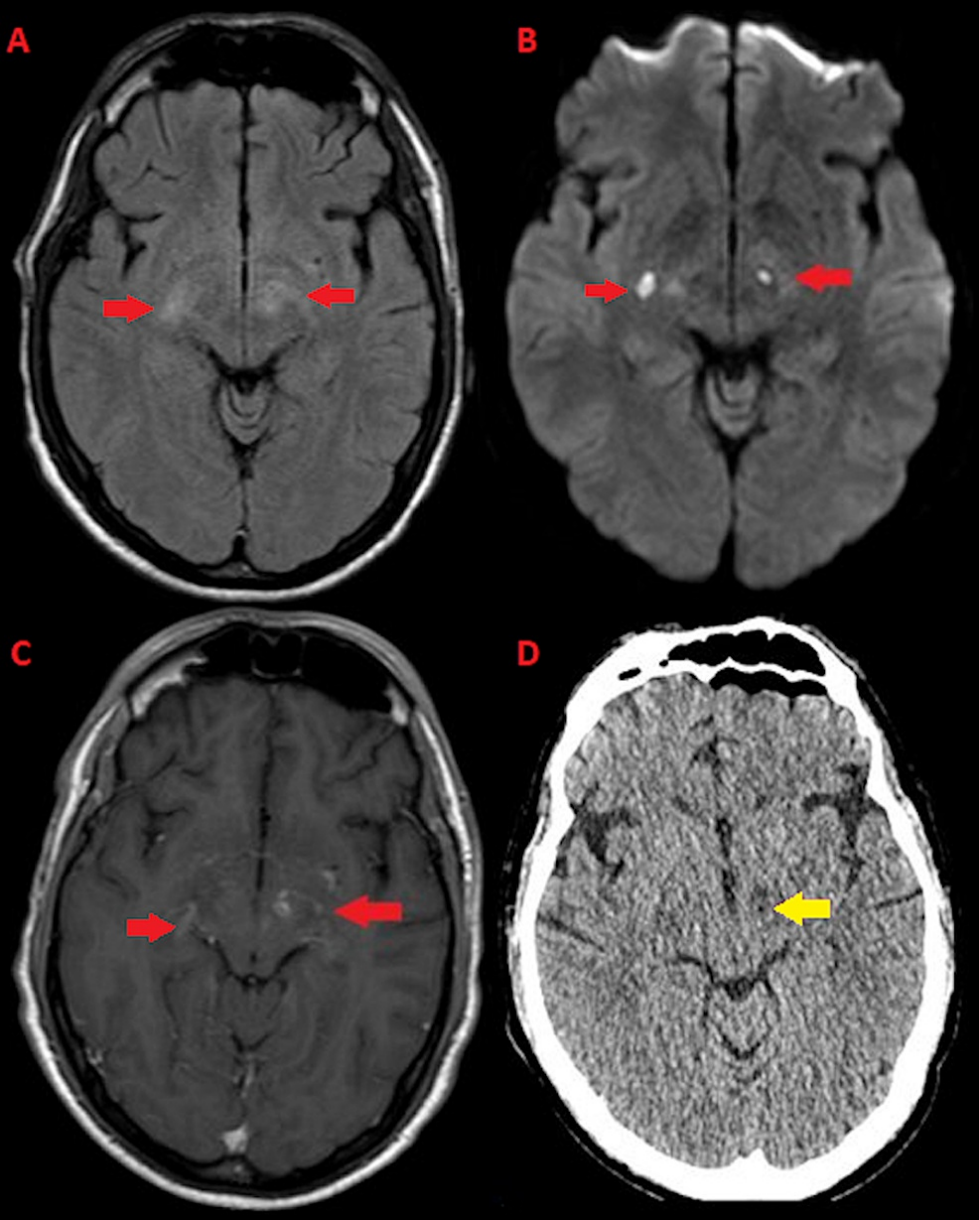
Magnetic resonance imaging of the brain Brain MRI demonstrating abnormal T2-weighted-fluid-attenuated inversion recovery hyperintense signal (a) and restricted diffusion (b) with associated enhancement (c) in the globus pallidus and internal capsule, suggestive of bilateral abscesses (red arrows). Post-surgical CT (d) with evidence of successful left pallidotomy (yellow arrow).
